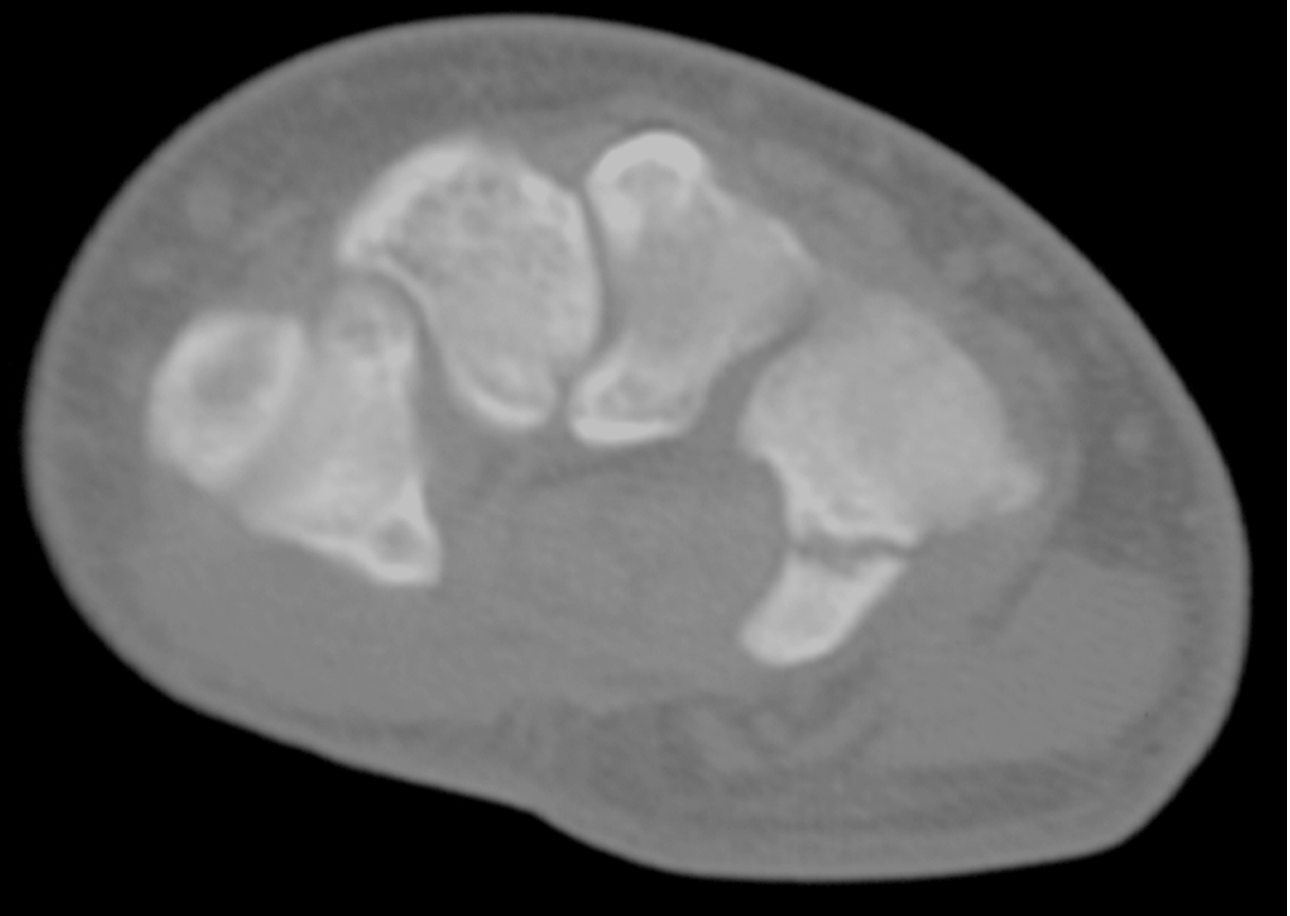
CT of right wrist indicating hamate hook fracture.
